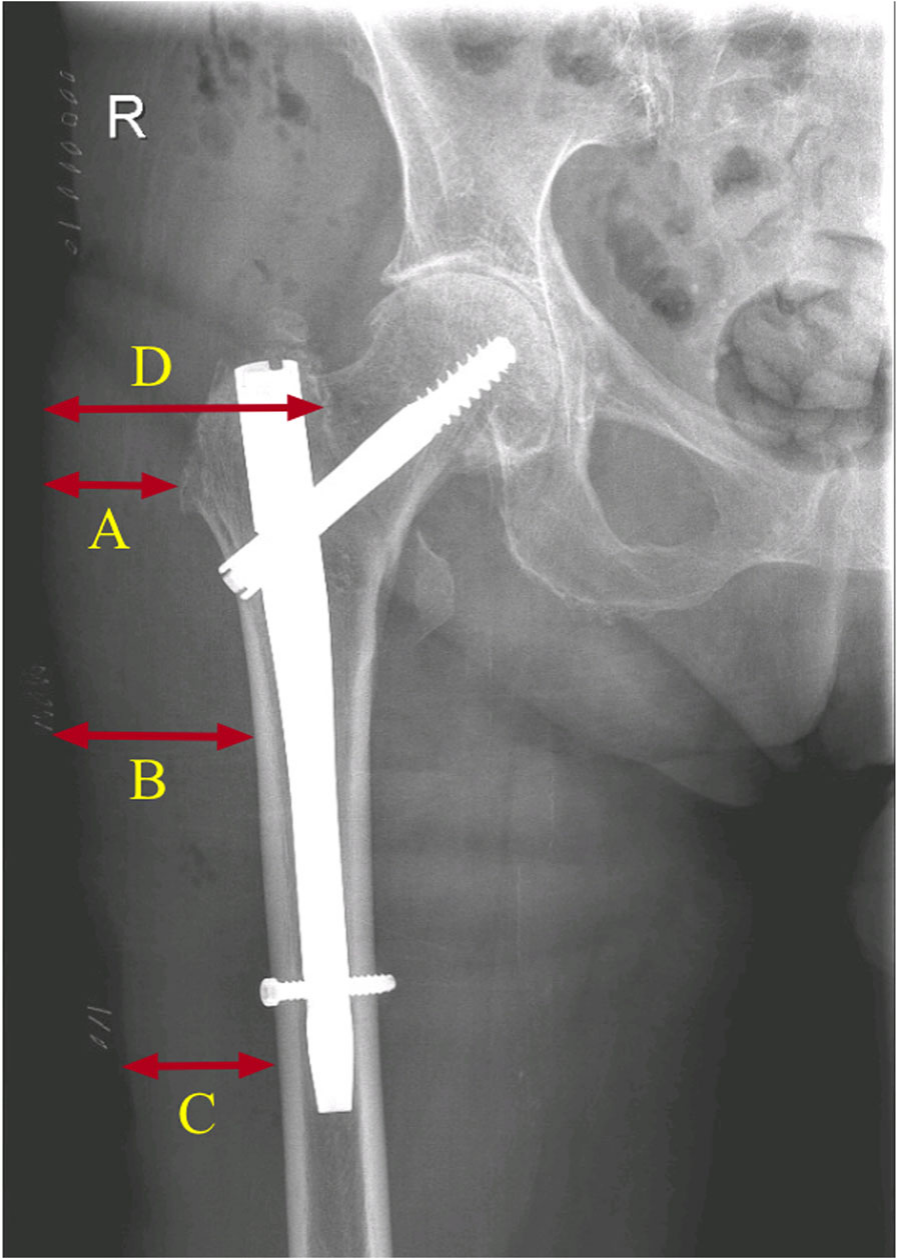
Tissue dimensions on an X-ray of an intramedullary hip nail for a right-sided peritrochanteric fracture. a Trochanter distance. b Mid femur distance. c Distal femur distance. d Shortest skin-to-fracture distance
